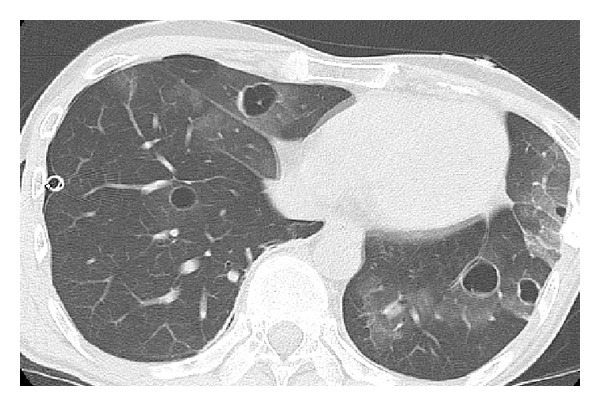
Chest computed tomography taken 9 months after the operation for pulmonary complications shows bilateral thin-walled cystic lesions that are surrounded by ground glass opacities, for which the patient underwent placement of a drainage tube in the pleural space for right pneumothorax.
